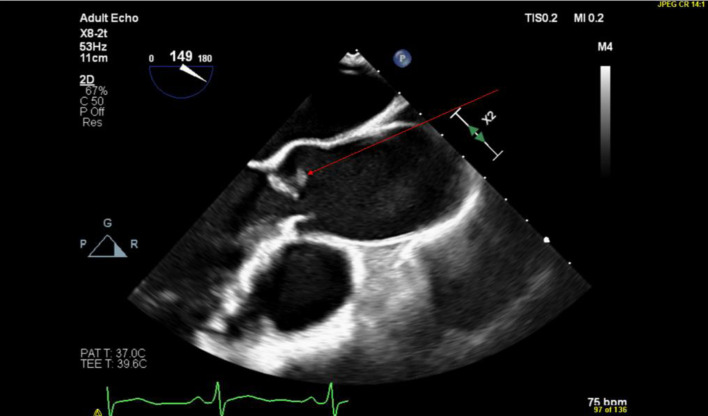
TEE showing a parasternal long axis view of the aortic valve with the papillary fibroelastoma indicated by the red arrow. TEE: transesophageal echocardiogram.
